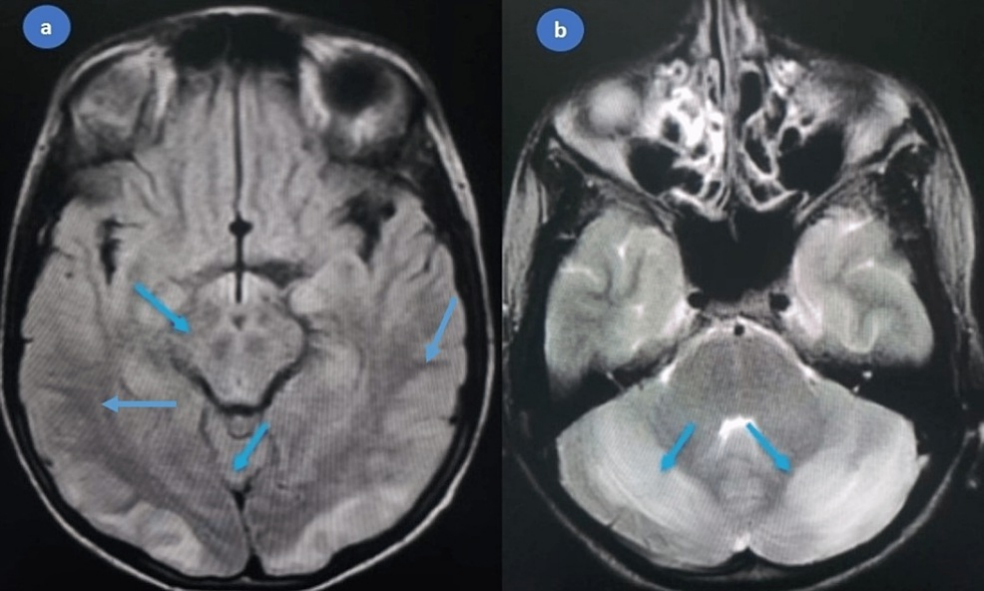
(a) MRI demonstrating hyperintensity on FLAIR, (b) T2 images involving bilateral parieto-occipital and medullary regions, mainly involving the cortical and subcortical areas and cerebral peduncles FLAIR: fluid-attenuated inversion recovery
